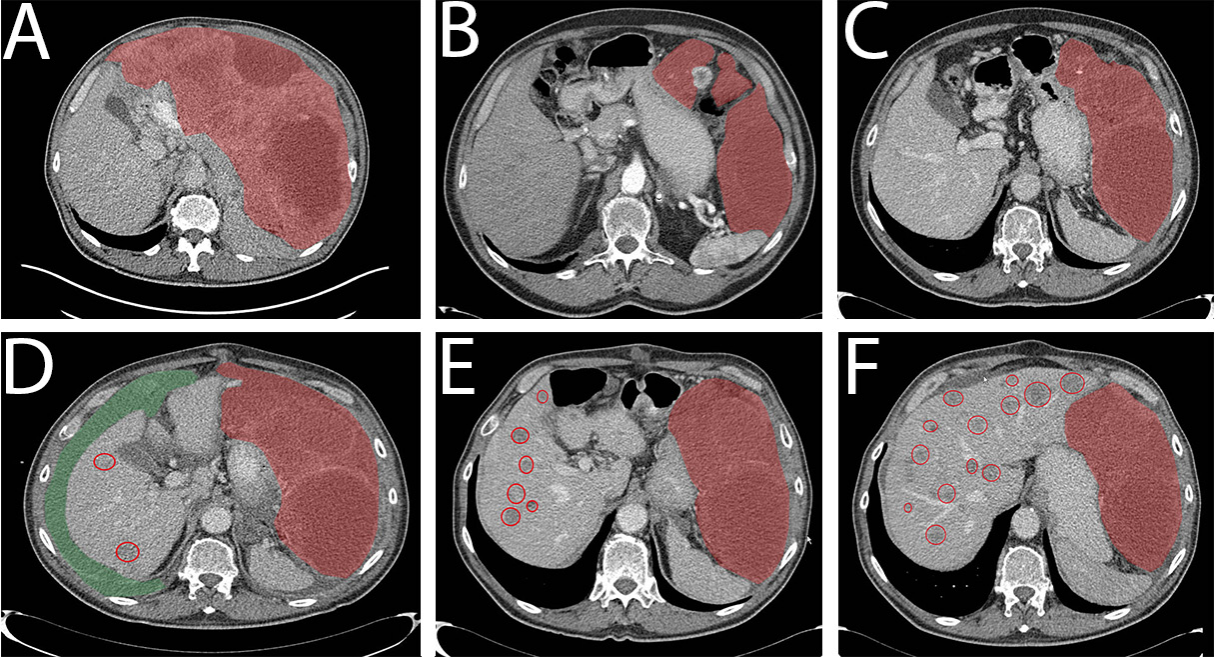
CT images of patient 1 Primary GIST (red area), liver metastases (red circles) and ascites (green) are indicated. A. Pre-treatment scan. B. After 12 months imatinib treatment, growing nodule. Start sunitinib. C. 6 months on sunitinib, stable disease. D. One year sunitinib. Progression. Ascites, liver metastasis. Start regorafenib. E. 3 months regorafenib, stable disease. Demarquation of the liver metastasis is seen as response on therapy. F. 6 months regorafenib, progression, more liver metastases are seen.
